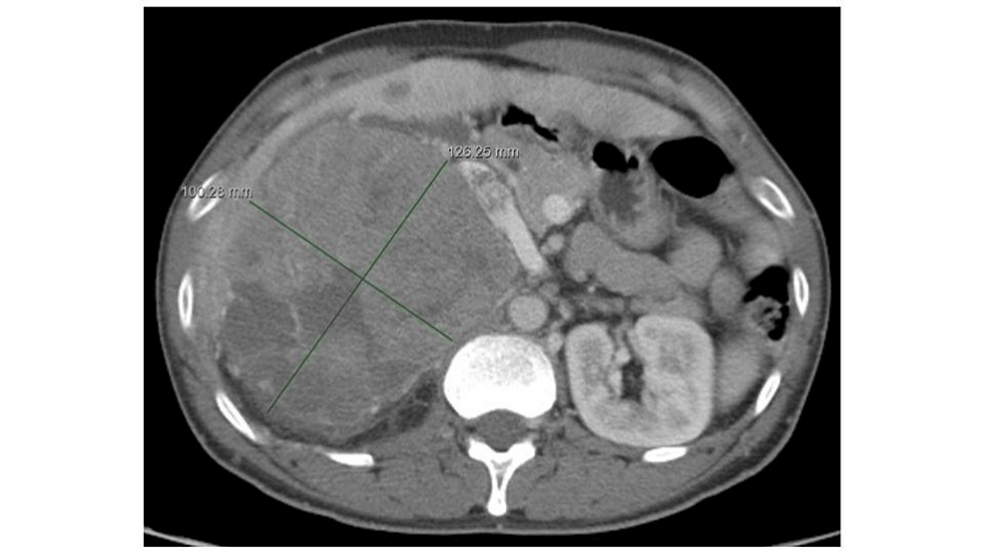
Axial view of the CT scan demonstrates a large right abdominal heterogeneous enhancing mass measuring 10.0 cm x 12.6 cm in length and width.
